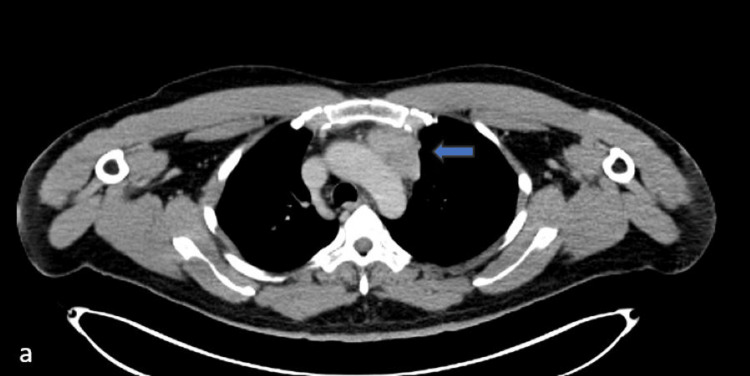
Axial contrast-enhanced computed tomography (CECT) image of the chest at the level of the aortic arch
